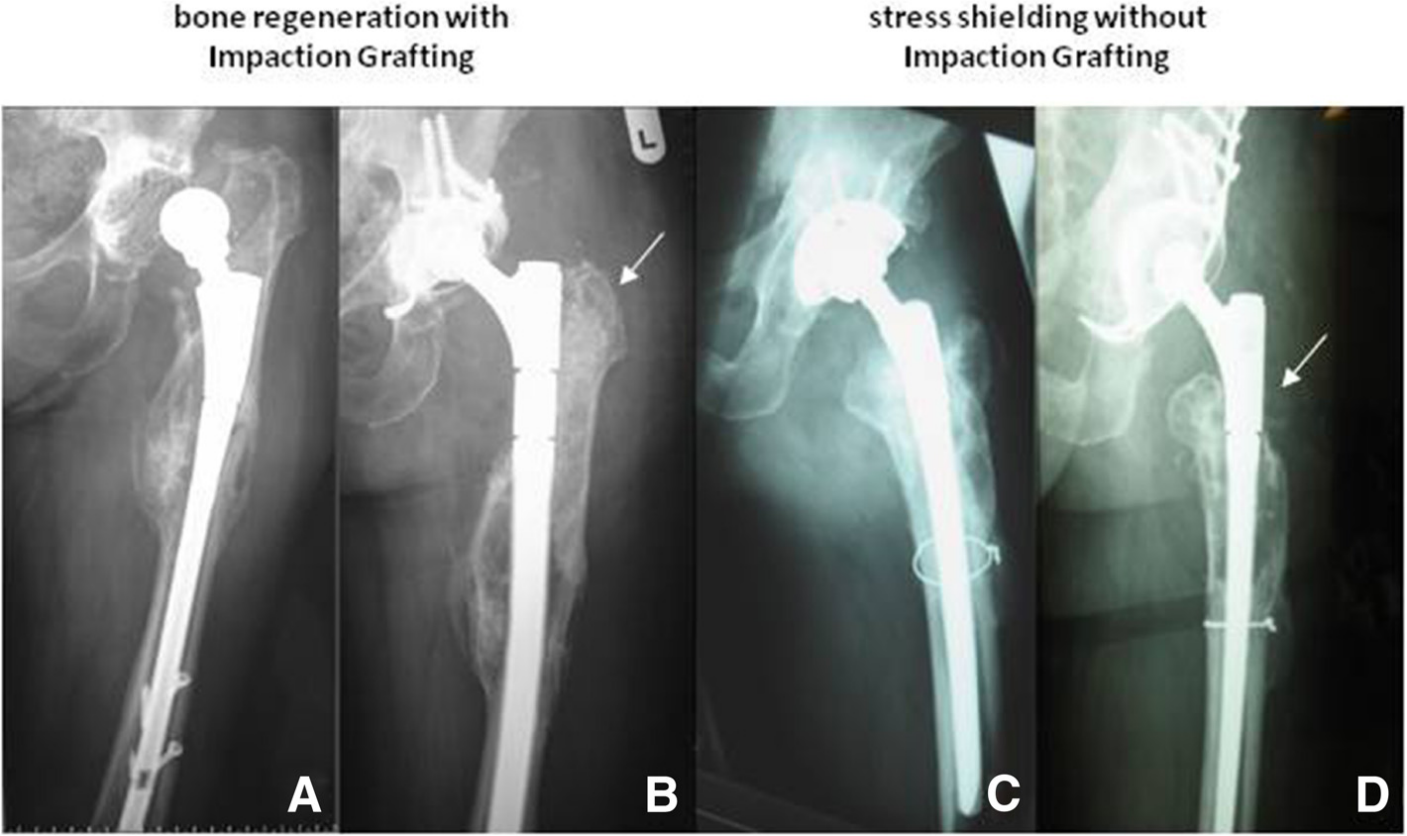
Radiographic assessment. Preoperative and two years postoperative follow-up radiographs of revisions with implantation of a MRP-TITAN stem with distal diaphyseal fixation. 3.A: preoperatively, 3.B: postoperatively with metaphyseal defect augmentation by impaction bone grafting. 3.B. shows consolidation of the metaphyseal allograft after impaction grafting. 3.C: preoperatively, 3.D: postoperatively without impaction bone grafting and stress shielding in the proximal femur.
